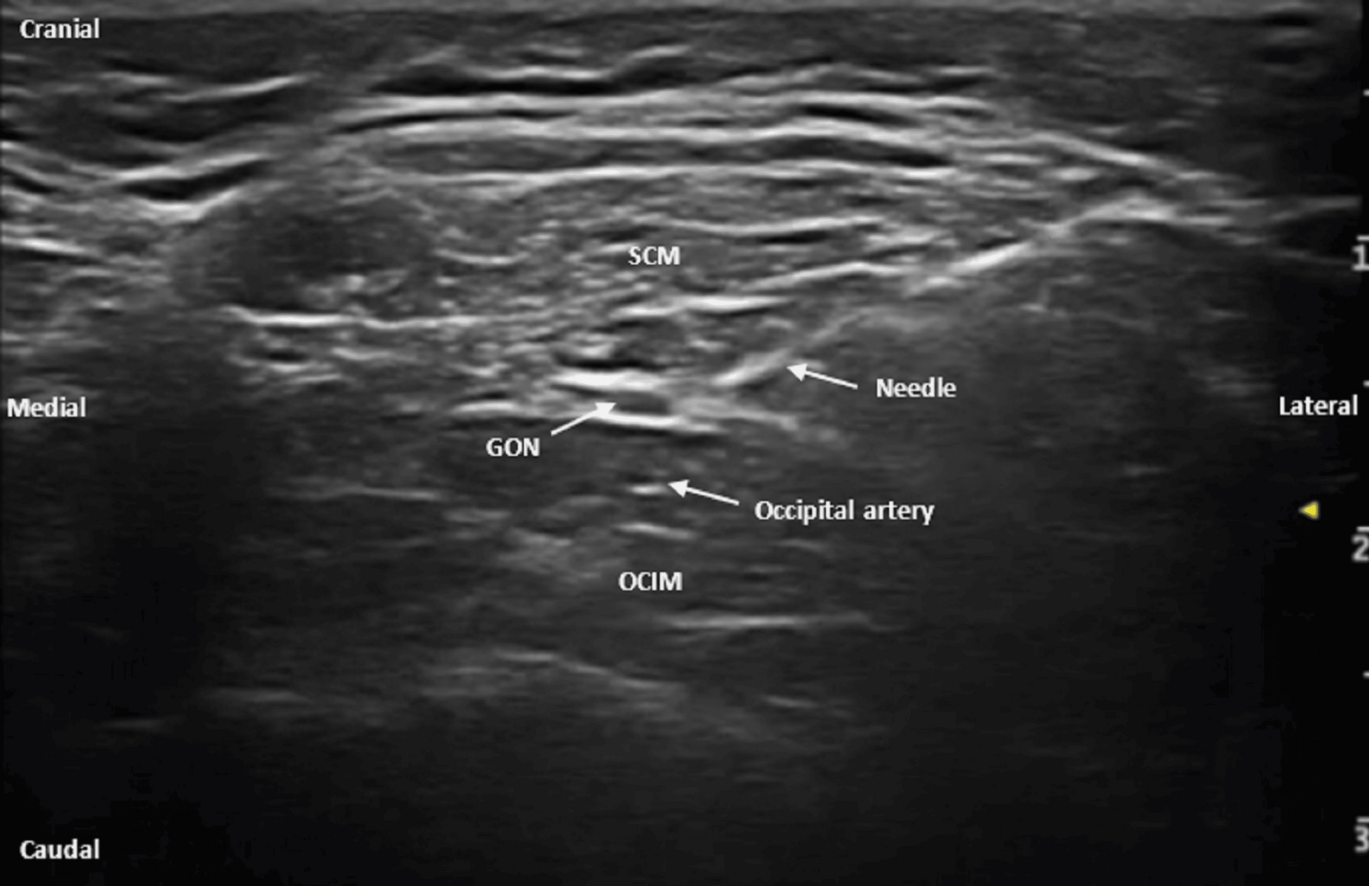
Ultrasound image of greater occipital nerve block SCM: semispinalis capitis muscles; GON: greater occipital nerve; OCIM: obliquus capitis inferior muscle
